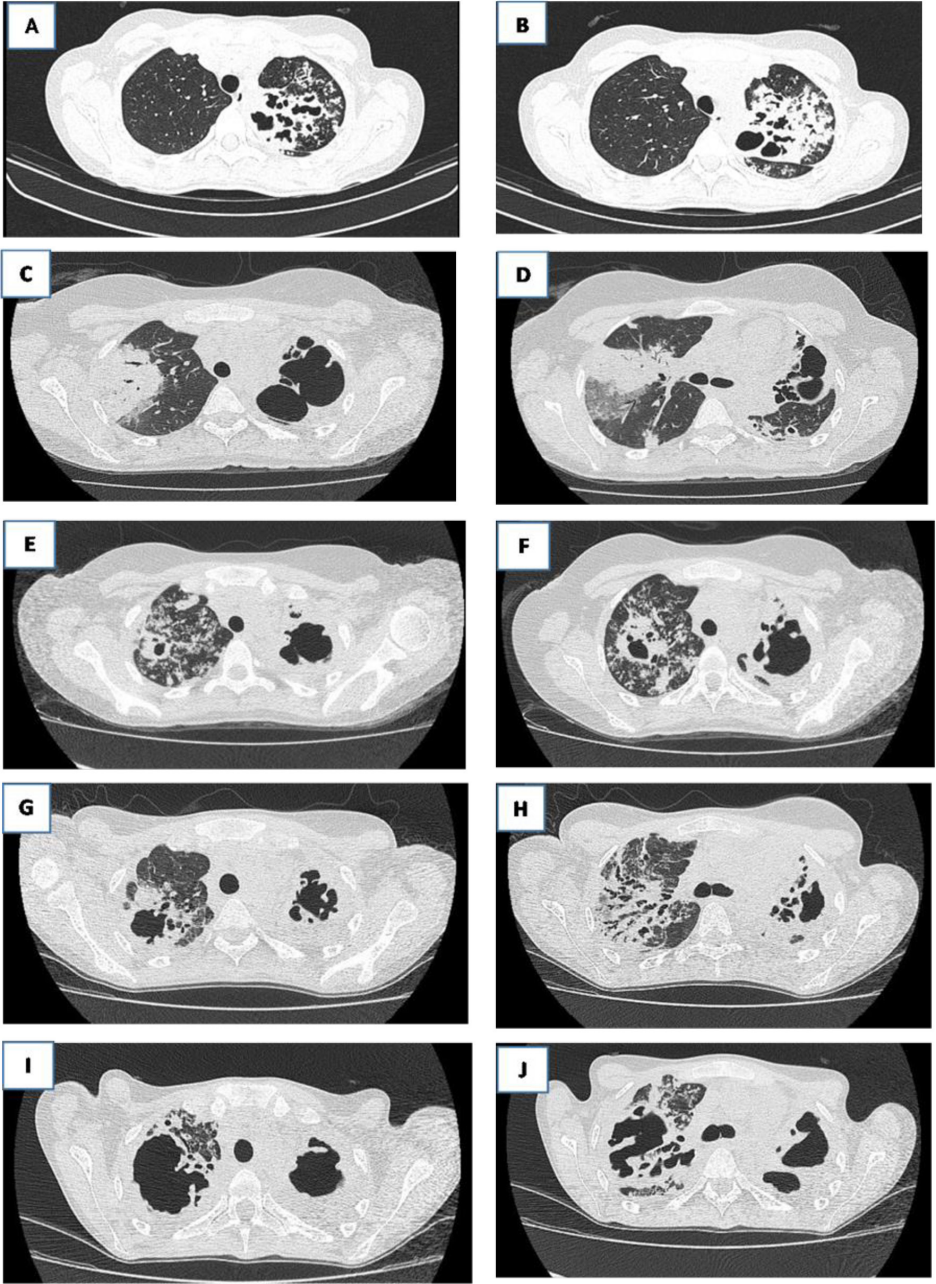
The evolution of chest CT scan images during treatment shows extensive pulmonary involvement: A and B) January 2017; C and D) September 2018; E and F) October 2019; G and H) June 2021; I and J) January 2022.
